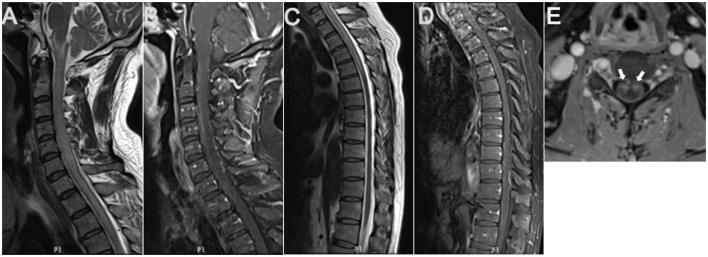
Cervicothoracic MRI. Sagittal cervicothoracic MRI T2-weighted images revealed longitudinal swelling of the medulla oblongata and cervicothoracic spinal cord extending to T6 level (A, C), with apparent abnormal flow void signals in the anterior surface of the cervical spinal cord (A). Contrasted MRI showed anterior and posterior perimedullary dilated tortuous veins from the cervical to T12 level (B, D), apparent enhancement in cervical spinal anterior horns on both sides (arrows) (E), and patchy enhancement in the whole cervicothoracic spinal cord (B, D).
